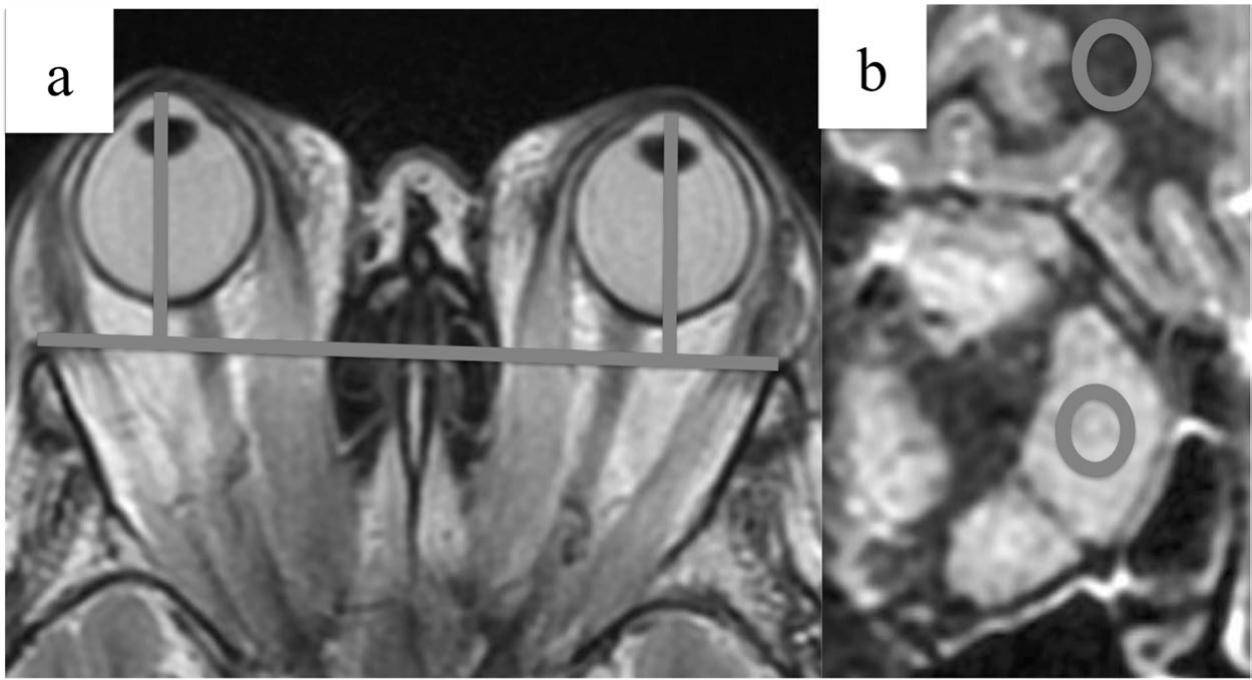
Measurement of proptosis and SIR. (a) Proptosis was defined as the distance between the corneal eminence and the connecting line of both zygomatic bones in an axial section T2WI. (b) SIR was defined as the ratio of enlarged muscular signal intensity to cerebral white matter in the STIR image. SIR, signal intensity ratio; STIR, short-tau inversion recovery; T2WI, T2-weighted image.
